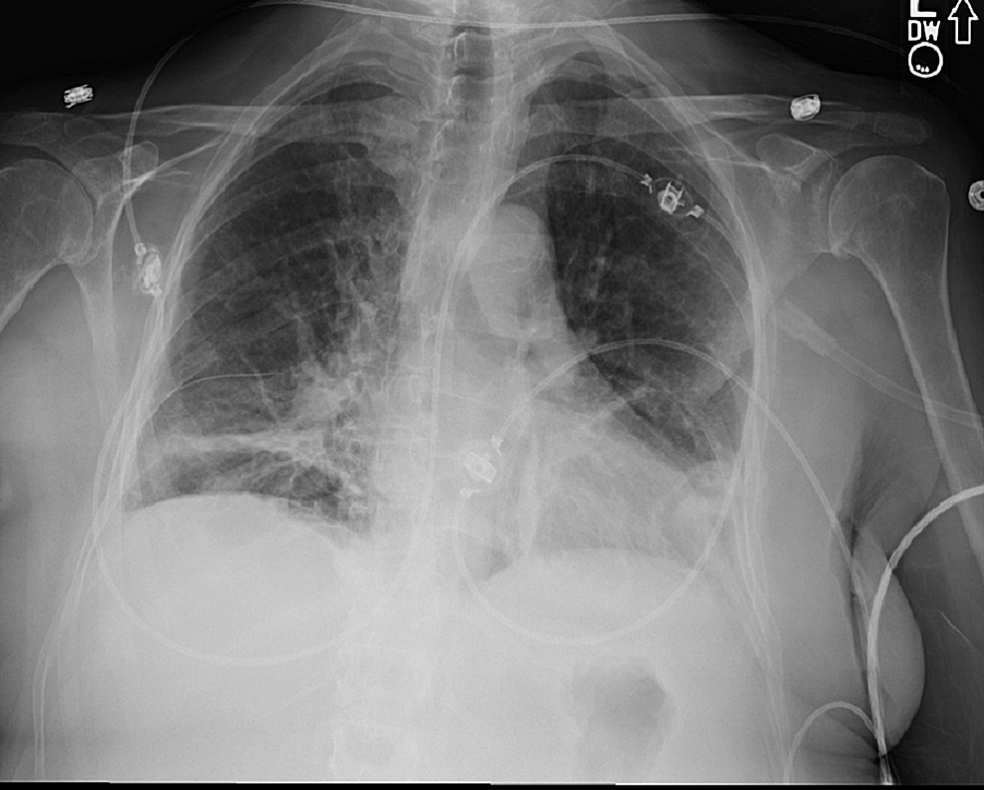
Chest radiograph on admission showing right perihilar and basilar airspace opacities concerning for pneumonia
